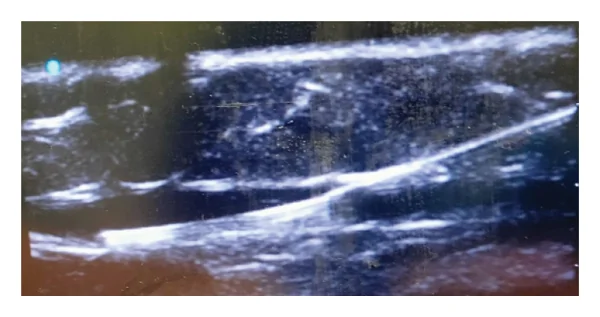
Insertion of the guidewire into the right great saphenous vein under ultrasound guidance.
